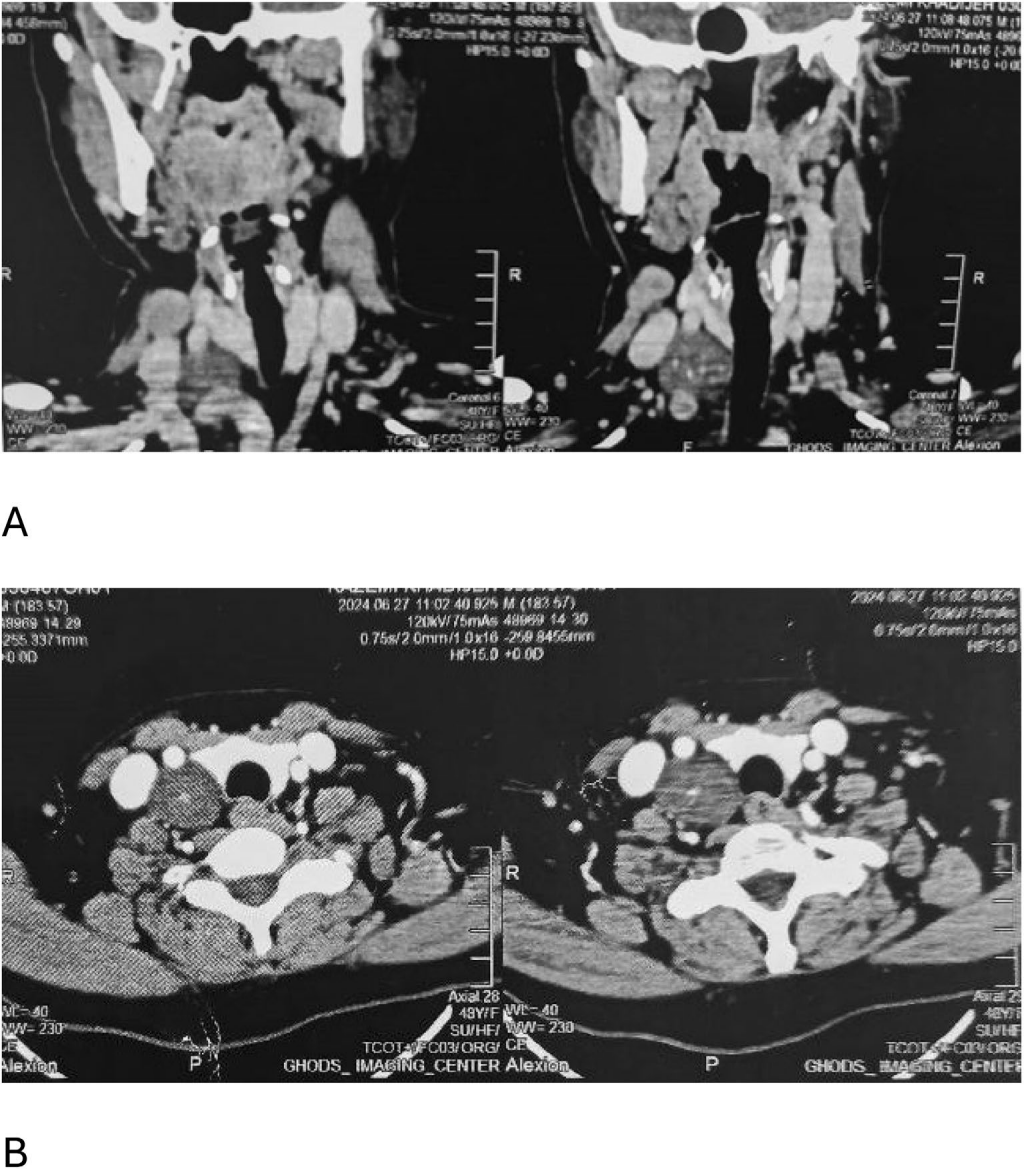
(A) Preoperative coronal CT scan with intravenous contrast showing a right‐sided hypodense and heterogenous mass (B) Preoperative axial CT scan.
